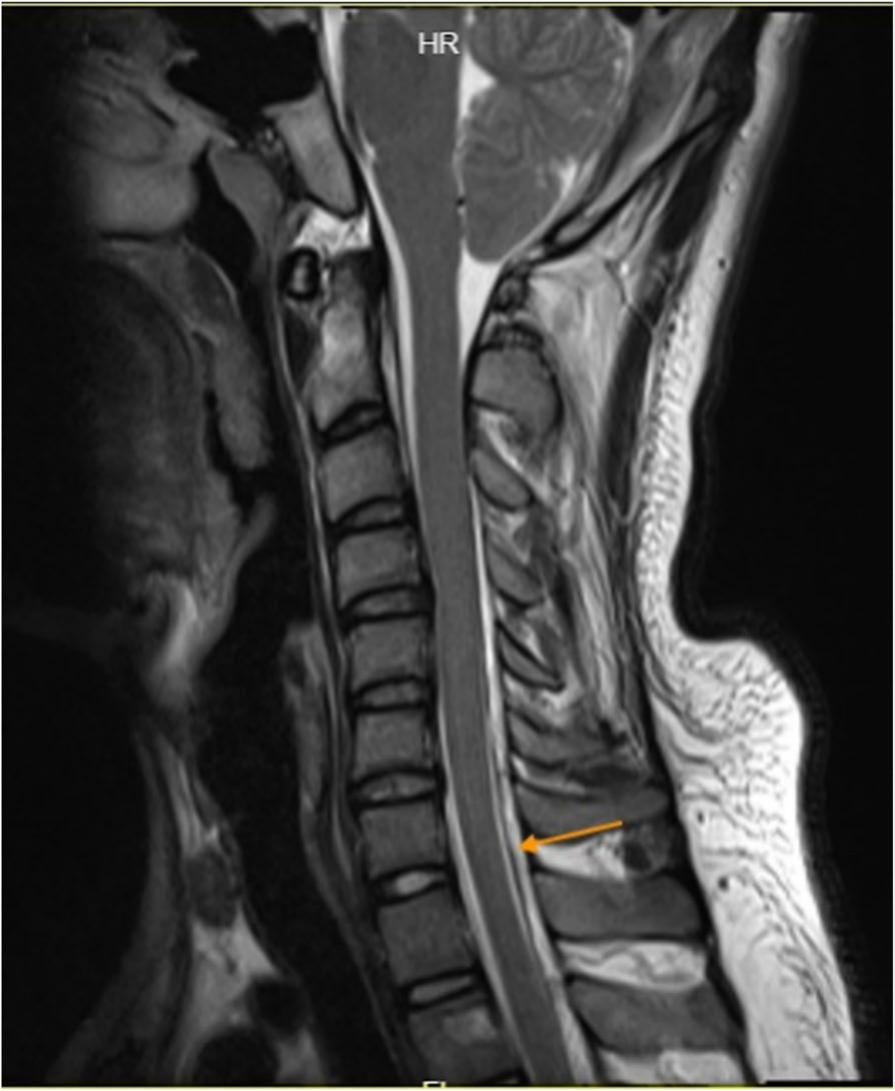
Sagittal T2-weighted MR image of the total spine showing posterior epidural fluid collections in the cervical and upper thoracic segments
